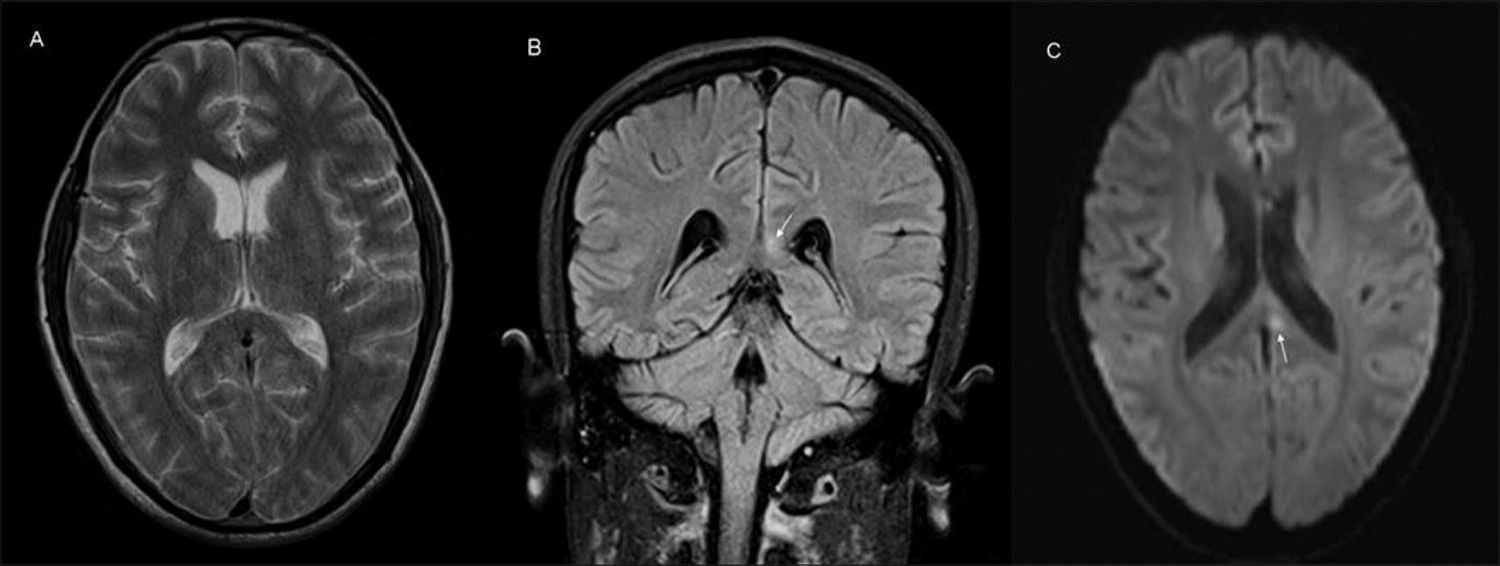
Axial T2W (A), coronal FLAIR (B), and diffusion-weighted (C) images show mild persistent hyperintensity (white arrow) in the corpus callosum, which however is less than in Figure 3
